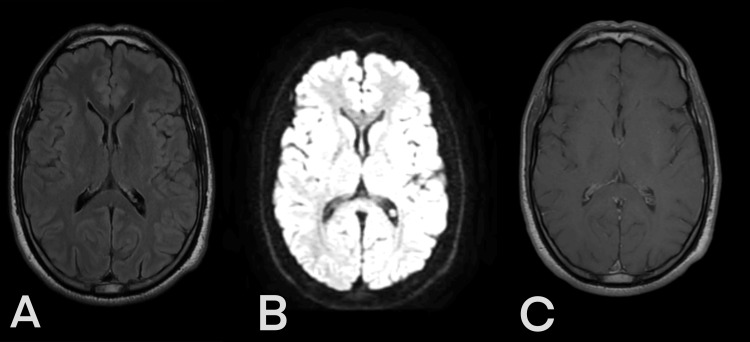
Brain MRI (axial view) obtained two months after the onset of the condition (A) Fluid‐attenuated inversion recovery image (FLAIR); (B) Diffusion‐weighted image (DWI); (C) T1‐weighted imaging
